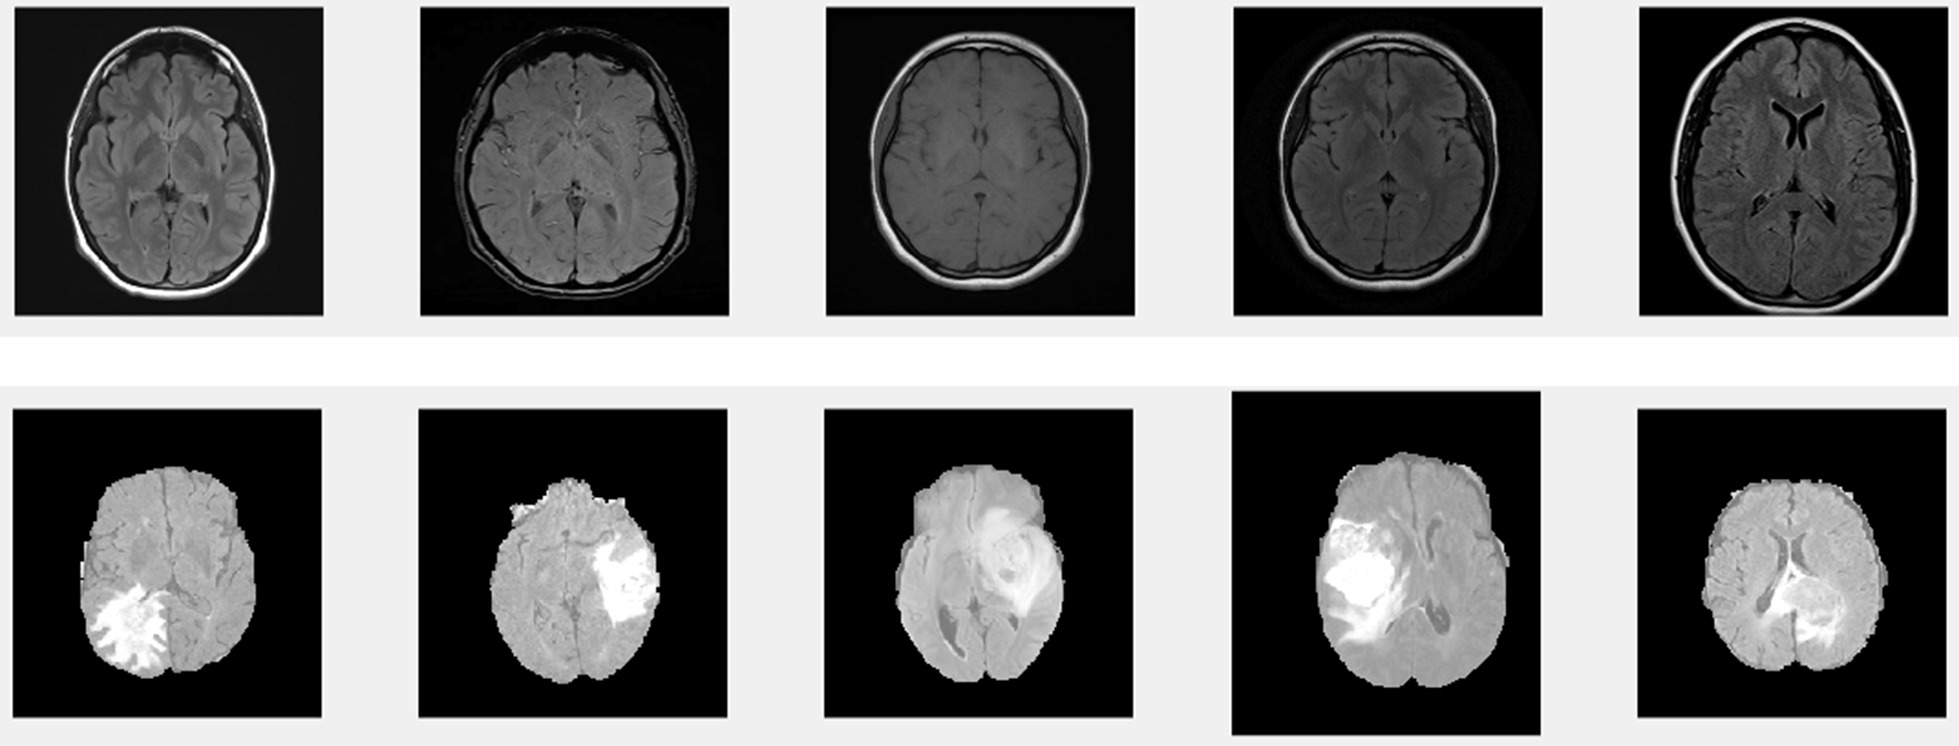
Top row: example of normal MRI images from HCP data set. Bottom row: brain tumor images from BRATS 2015 datset
